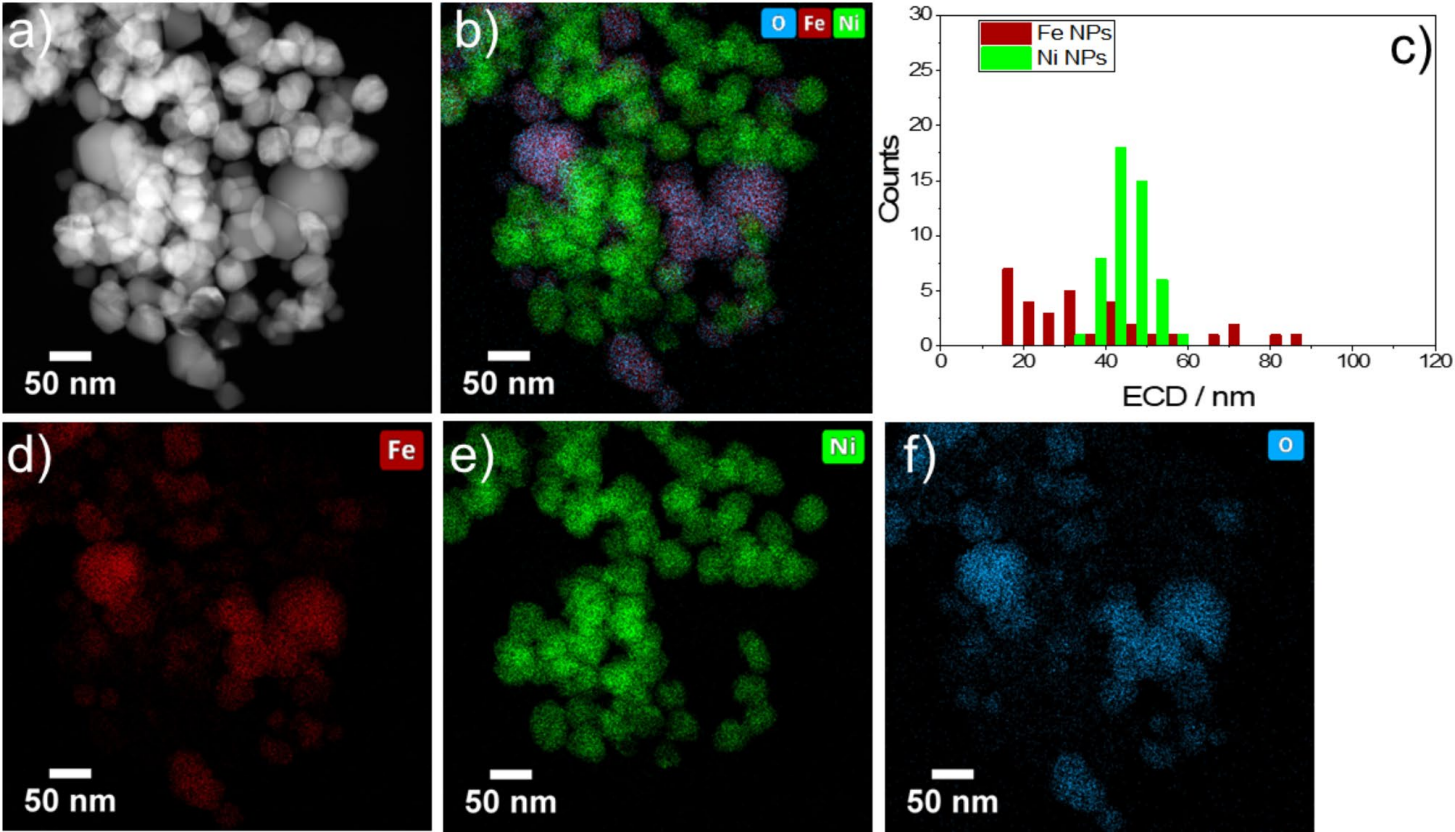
Fe: Ni = 2:3 NPs: (a) HAADF-STEM image, (b) EDS elemental mapping of O K, Fe K and Ni K X-ray lines, (c) Histogram of the size distribution of Fe and Ni NPs as detected by EDS in (b), separate EDS elemental mappings of Fe (d), Ni (e), and O (f).
